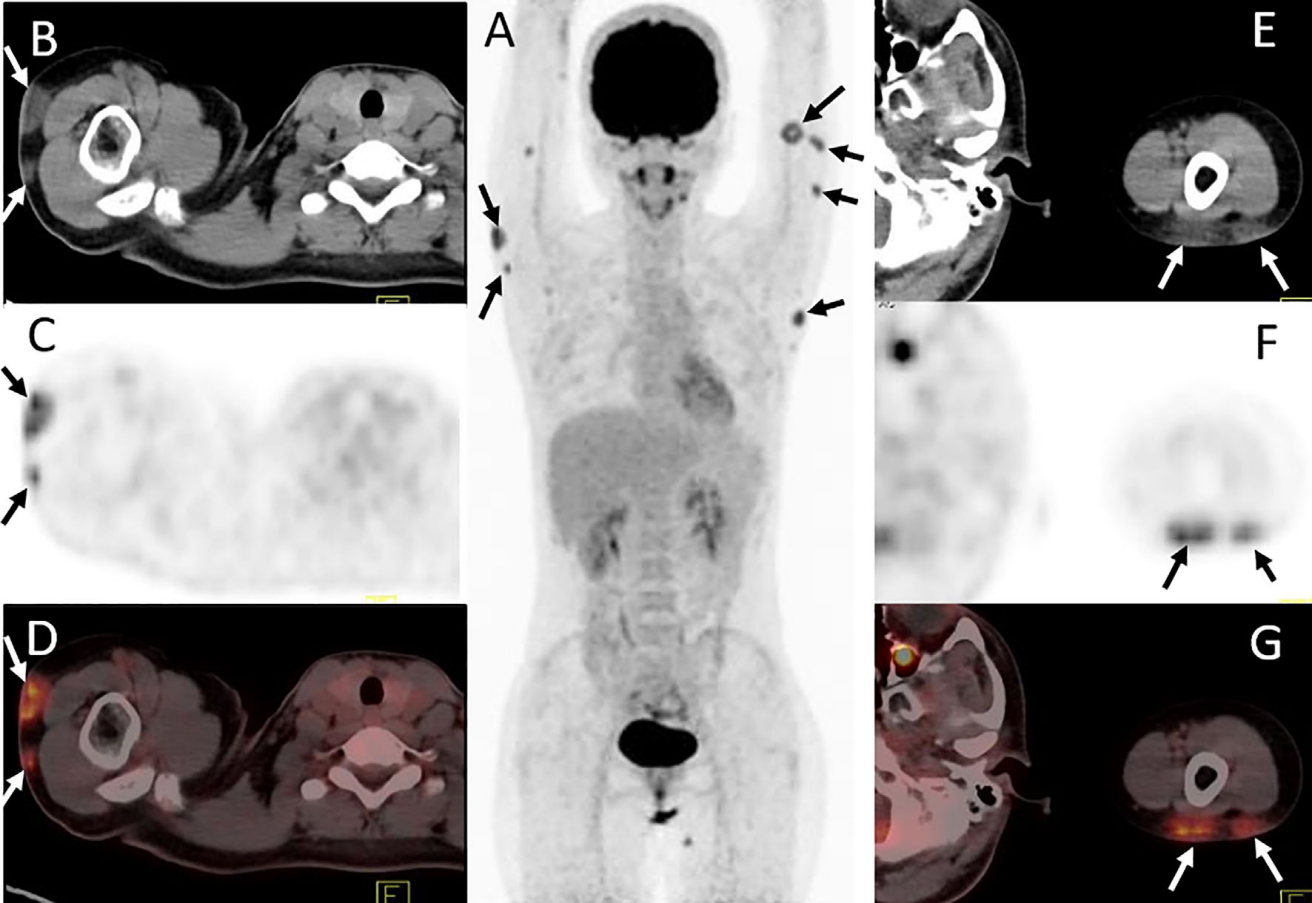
Twenty-four-year-old female with subcutaneous panniculitis-like T-cell lymphoma. Three-dimensional maximum intensity projection image (A), axial CT (B, E), corresponding PET (C, F) and fusion PET/CT (D, G) images from ^18^F-FDG PET/CT clearly show multiple intensely ^18^F-FDG-avid subcutaneous infiltrative nodules in both upper arms with poor or well defined borders (arrows), representing sites of lymphoma.
